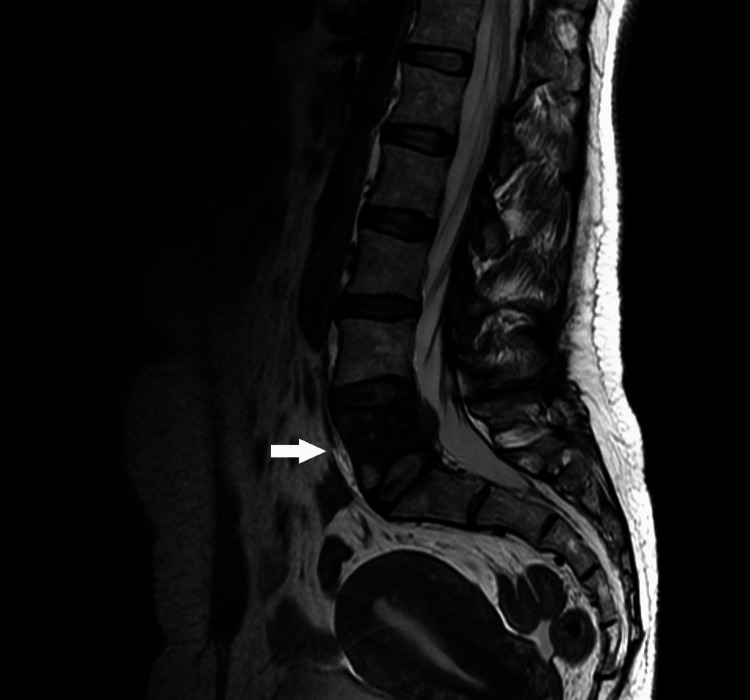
MRI, T2WI sequence. On MRI, the lesion was isointense on T2WI.
